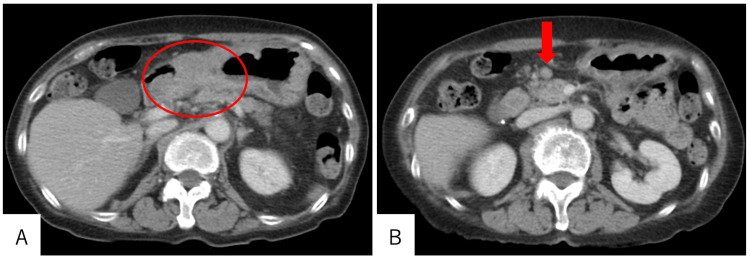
(A-B) Enhanced computed tomography finding demonstrating wall thickening of the gastric antrum (red circle) and significant lymph node enlargement near the pyloric ring (red arrow).
